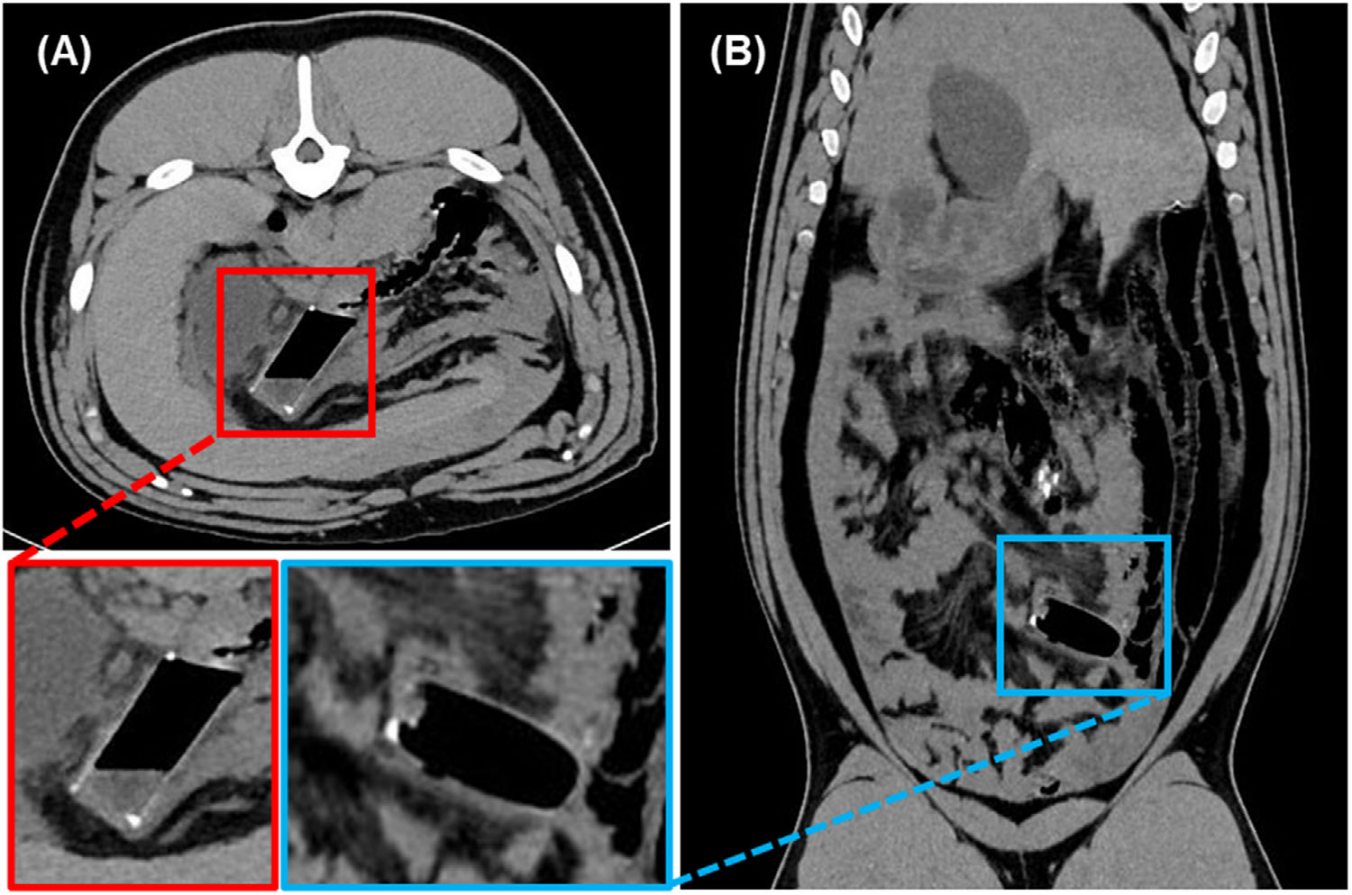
Representative CT images showing stents one‐week post‐placement. (A) Transverse view. (B) Coronal view. Observation of vascular clips confirms that the stent did not migrate from their initial positions.
